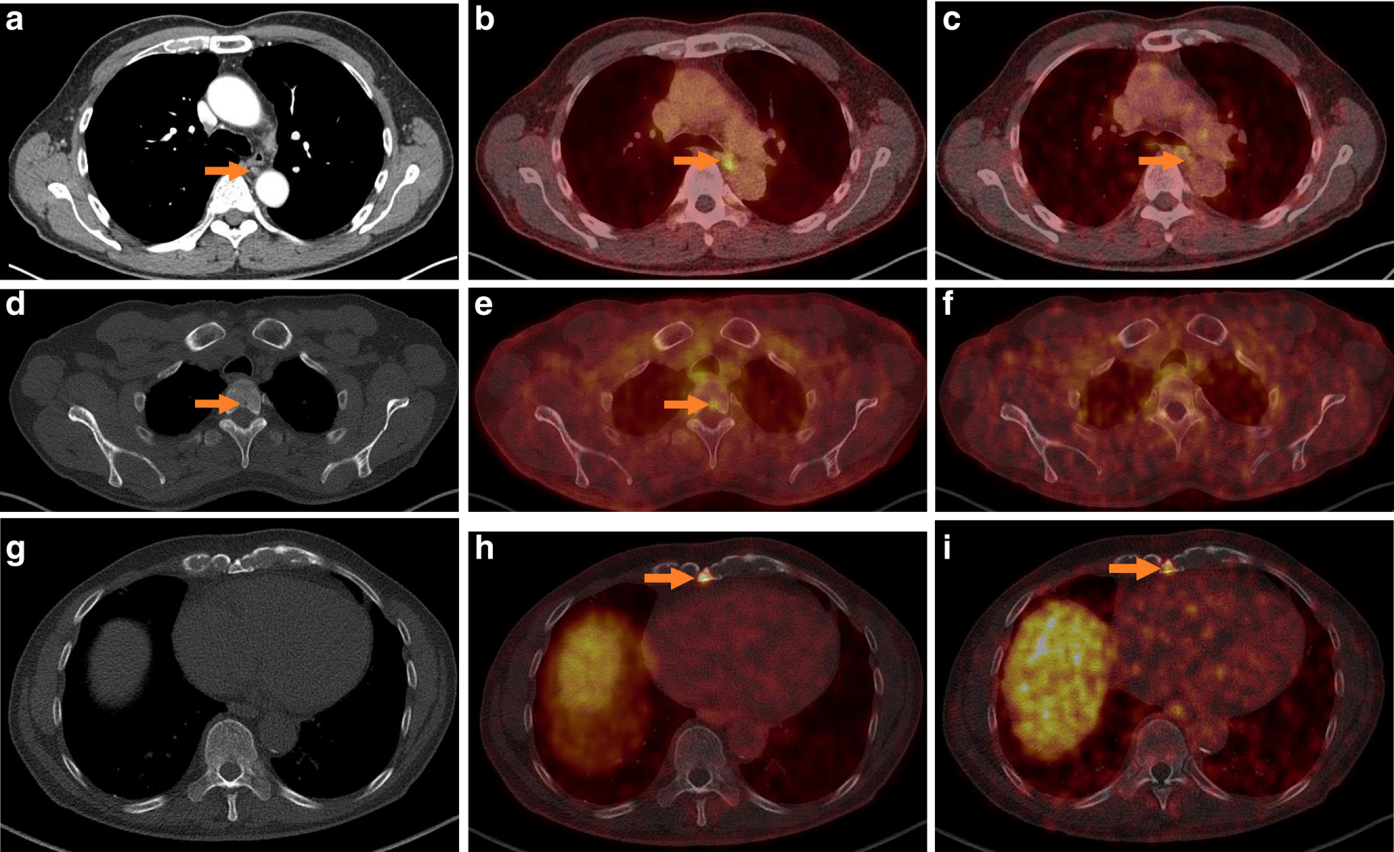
Patient no. 12, Biograph mCT 128. [^18^F]DCFPyL PET/CT (b) was suspicious of an additional lymph node metastasis in the dorsal mediastinum, visible as a normal-sized lymph node in a low-dose CT scan without tracer accumulation on c [^68^Ga]Ga-PSMA-HBED-CC PET/CT. d Low-dose CT in bone window and e [^18^F]DCFPyL PET/CT were suspicious of a bone metastasis in vertebra Th3, barely detectable in f [^68^Ga]Ga-PSMA-HBED-CC PET/CT. h [^18^F]DCFPyL PET/CT was clearly suspicious of bone metastasis in the *processus xiphoideus sterni*, hard to detect in g low-dose CT or i [^68^Ga]Ga-PSMA-HBED-CC PET/CT.
